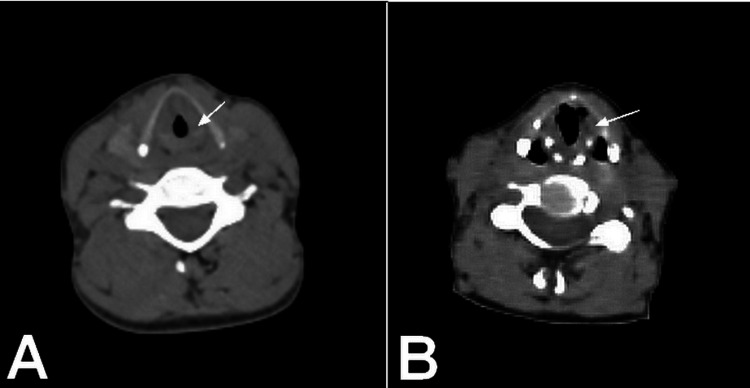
A. Axial CT scan showing a soft-tissue lesion involving the left vocal cord with medial protrusion resulting in partial narrowing of the laryngeal airway (white arrow). B. Follow-up axial CT scan demonstrating marked regression of the lesion after three years of methotrexate therapy, with improvement of the laryngeal airway caliber (white arrow).
